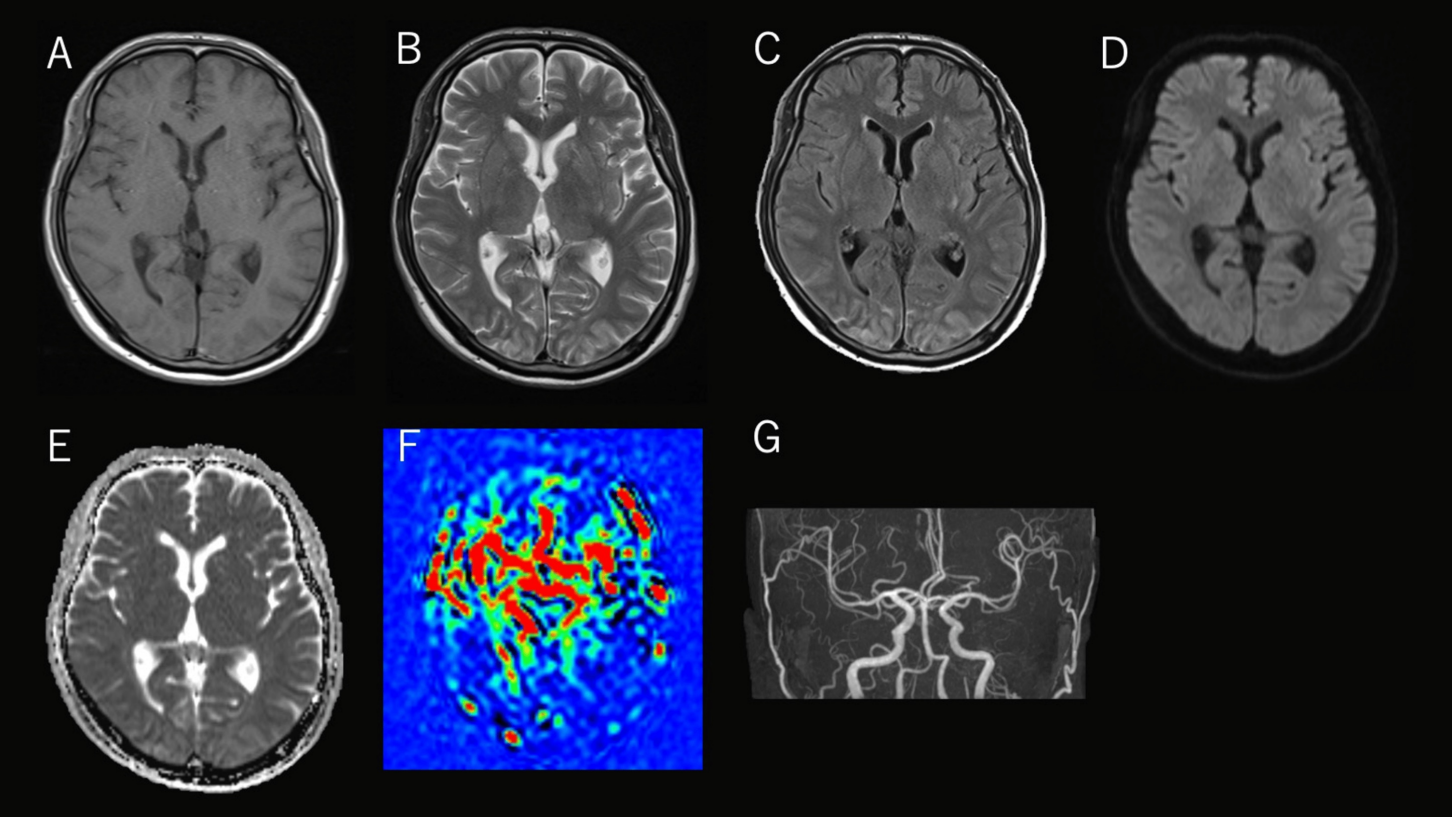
Brain MRI findings on the day of admission (A) T1-weighted images show no abnormal signal intensity. (B) T2-weighted and (C) FLAIR images demonstrate hyperintensity involving the cortex and subcortical white matter, predominantly in the bilateral parieto-occipital regions. (D) Diffusion-weighted imaging (DWI) shows no corresponding hyperintensity, and (E) the apparent diffusion coefficient (ADC) map shows increased signal without ADC reduction, indicating the absence of true diffusion restriction. (F) Arterial spin labeling (ASL) demonstrates hyperperfusion in the bilateral middle cerebral artery (MCA) and posterior cerebral artery (PCA) territories. (G) Magnetic resonance angiography (MRA) shows increased visualization/prominence of the bilateral anterior cerebral artery (ACA), MCA, and PCA. Overall, these findings are consistent with posterior reversible encephalopathy syndrome (PRES). Note: The left side of each image corresponds to the patient’s right side.
